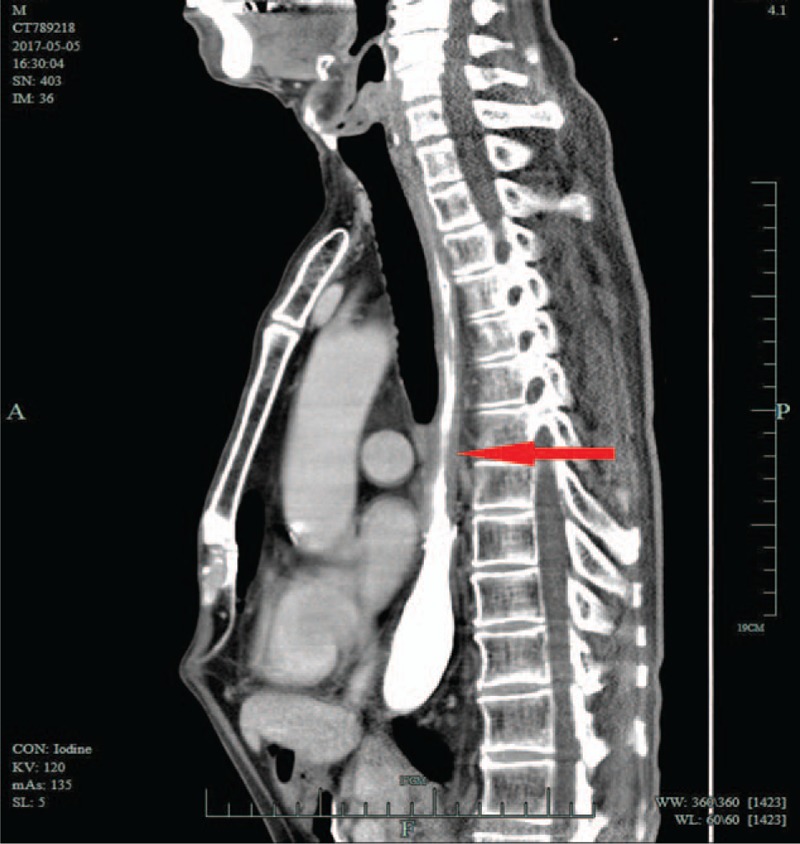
CT scanning at 31 months showed that the esophageal wall (arrow) remained thickened, similar to the findings from the esophageal CT scan 14 months prior (shown in Fig. 6). CT = computed tomography.
